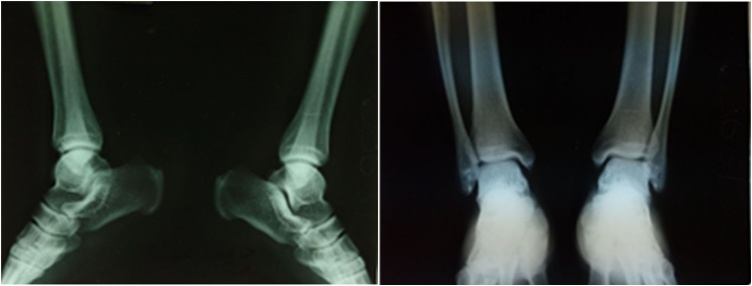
Pre-operative X-ray of both ankle.
